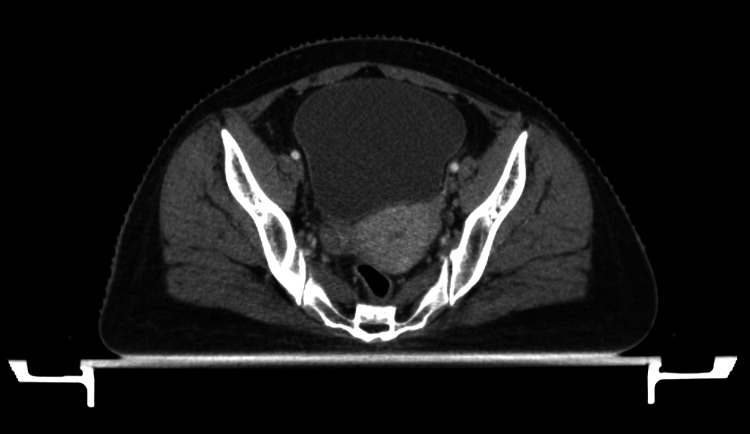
Response assessment planning CECT scan (axial section). CECT: contrast-enhanced computed tomography
